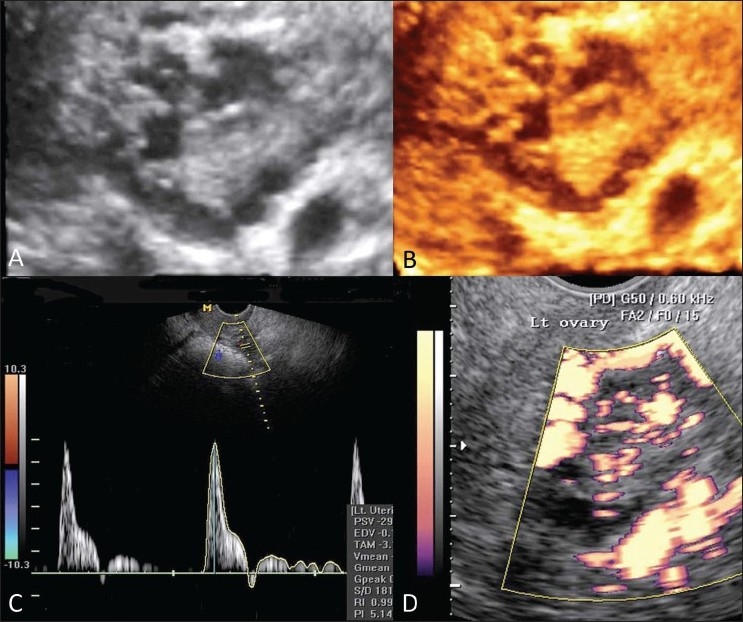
Polycystic ovary. Three-dimensional gray-scale (A) and color (B) images show a polycystic ovary. Spectral Doppler (C) and power Doppler (D) images show reduction in diastolic flow, reverse diastolic component, and increased stromal vascularity in polycystic ovary
